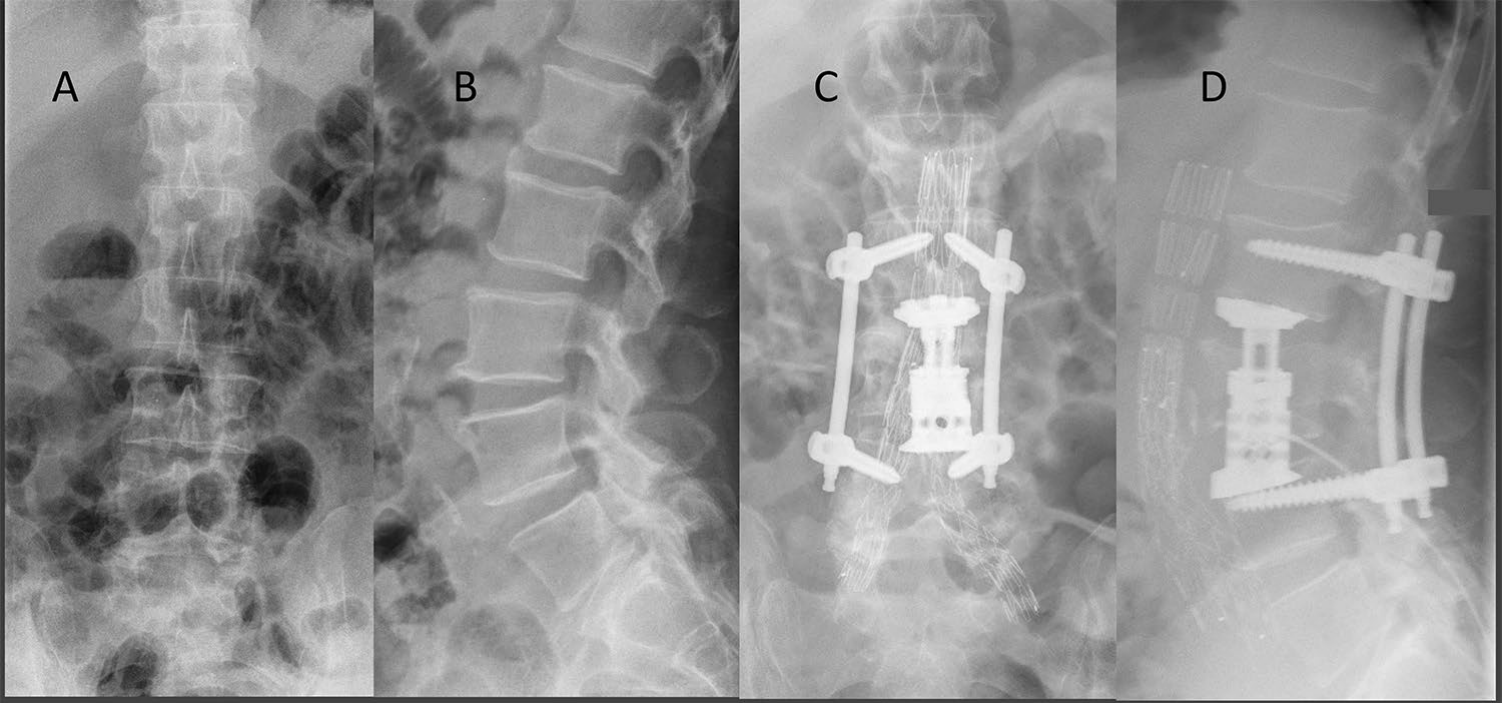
X-ray of patient 5 in the a anteroposterior and b lateral view at the beginning of clinical symptoms and after endovascular aortic repair (EVAR), posterior instrumentation and anterior vertebral body replacement
